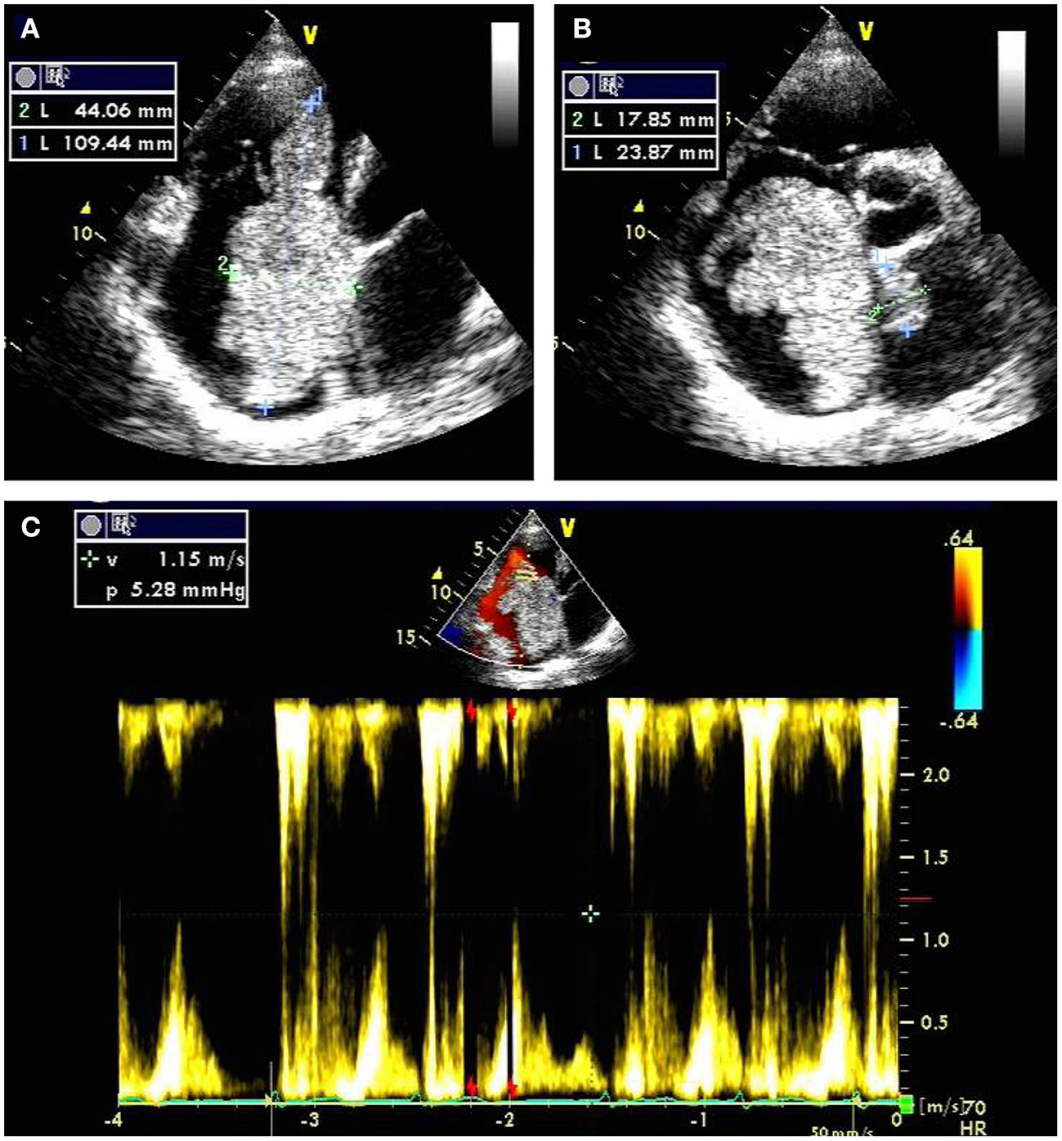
Transthoracic echocardiography preoperatively showing a giant biatrial mass with the size of 44.06 mm × 109.44 mm in the right atrium (A) and 17.85 mm × 23.87 mm in the left atrium (B); Color Doppler flow imaging showing severe mechanical hemodynamic obstacles in the right atrium primarily occupied by the giant tumor tissue (C).
